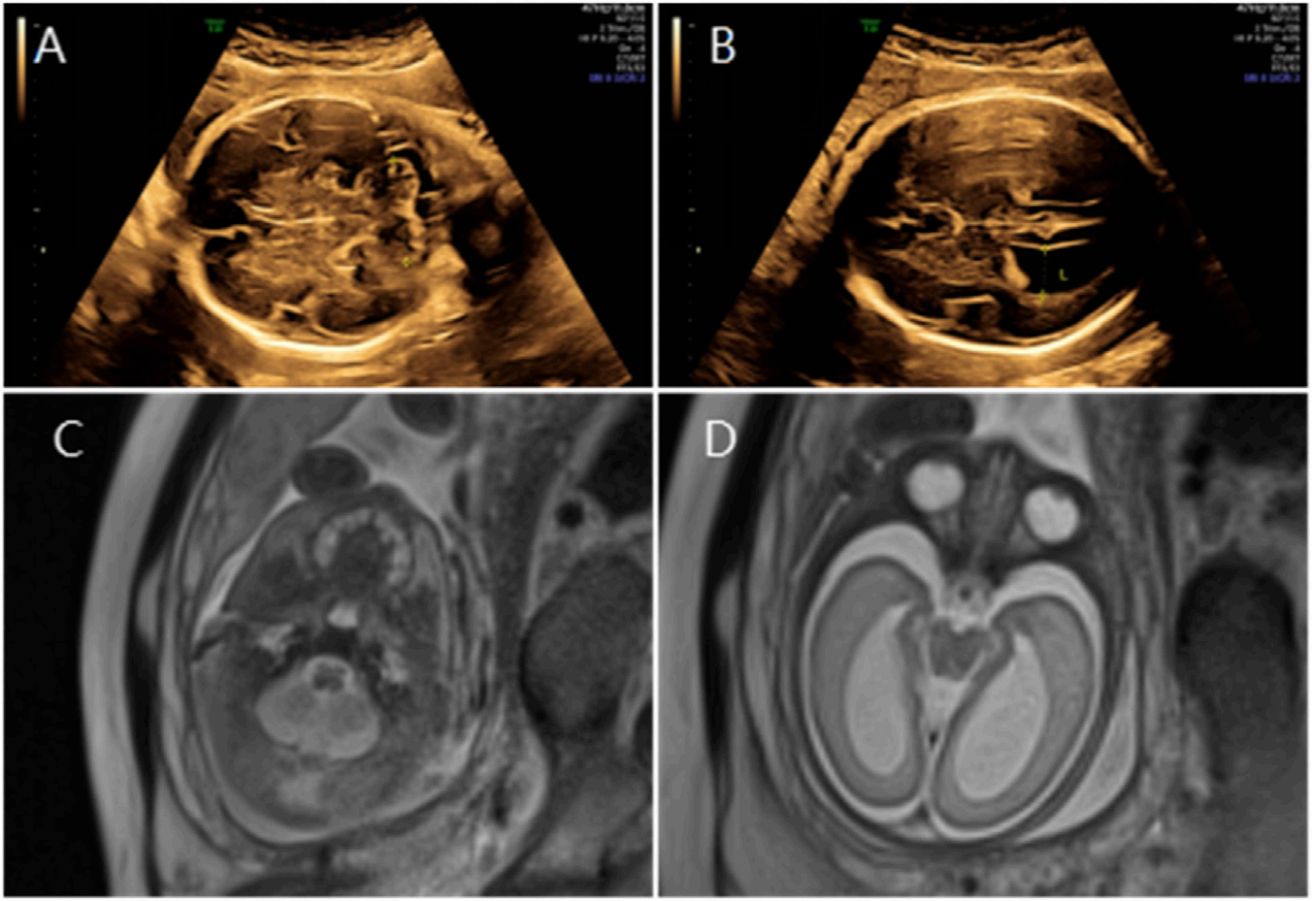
(A) Transverse ultrasound section showing the fetal cerebellum with a transverse cerebellar diameter of 27.8 mm (<–3.0 SD for gestational age). (B) Axial ultrasound view in thetransventricular plane demonstrating bilateral ventriculomegaly with atrial widths of 12.3 mm (left) and 12.5 mm (right). (C,D) Axial T2-weighted fetal MRI confirming reduced cerebellar size and bilateral ventriculomegaly.
